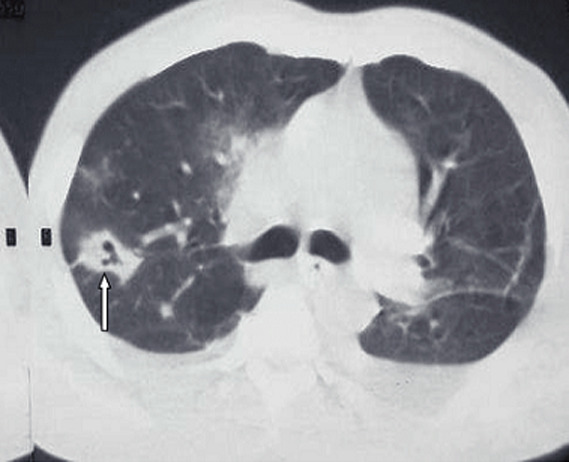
septic pulmonary embolus (arrow) chest CT scan
